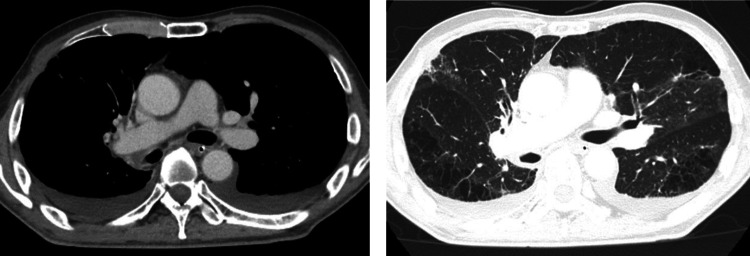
Chest CT 1 month after radiotherapy showed that atelectasis in right lung improved and the primary lung cancer shrank
